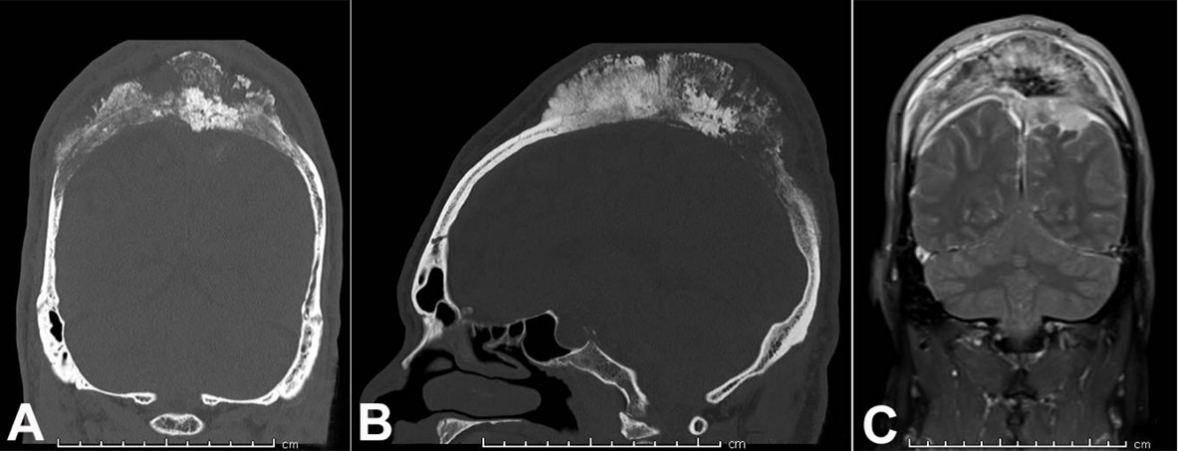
A and B (coronal and sagittal views respectively) – Bone computed tomography (CT). Large midline permeative calvarial lesion with areas of thickening/erosion of the inner table and extensive periosteal reaction; C – Coronal post-gadolinium fat-saturated T1 sequence. Large intraosseous mass with demonstration of intracranial extension, invasion into the superior sagittal sinus, and regional dural thickening and enhancement.
